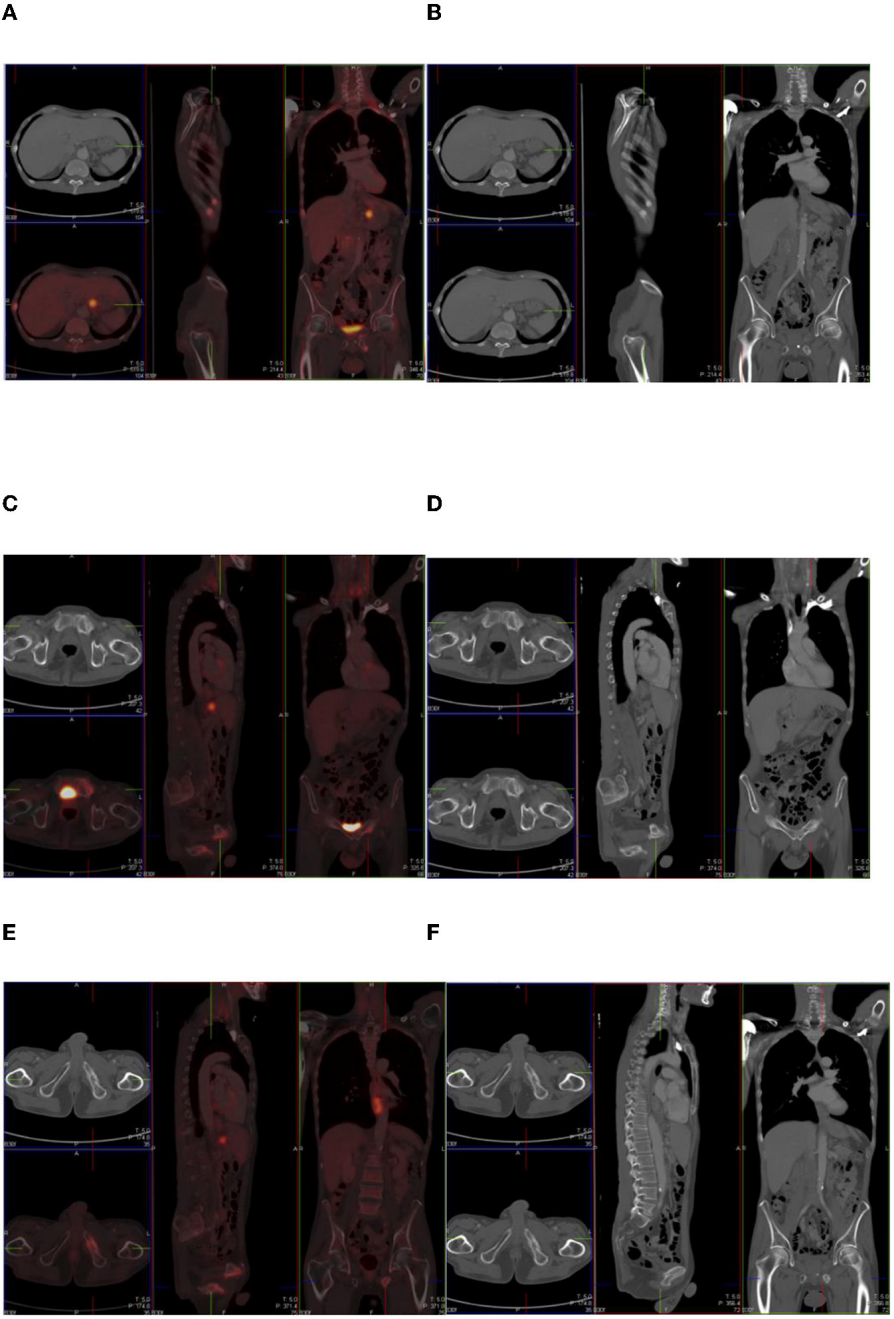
Combined PET/CT and CT images of a 54-year-old man with esophageal melanoma. He has multiple sites of skeletal uptake attributable to recent trauma: in the R lateral 8th and 9th ribs (A,B), superior pubic ramus (C,D), and inferior pubic ramus (E,F).
